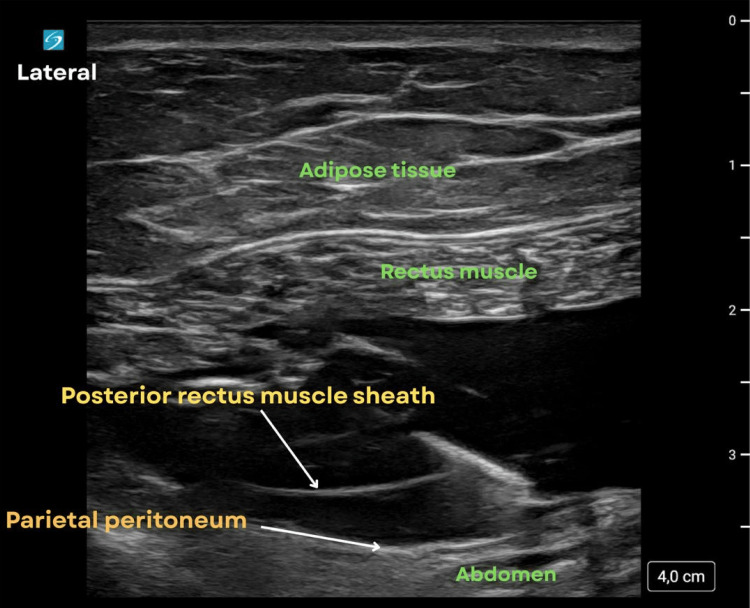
DRS block After an initial saline injection into the conventional rectus sheath plane to visualize the needle tip, the needle is advanced through the posterior rectus sheath into the preperitoneal plane. Injection of the local anesthetic opens the preperitoneal compartment, producing a characteristic V-shaped spread and displacing the parietal peritoneum deeper. Ultrasound orientation markers are shown within the image (lateral to the left, medial to the right; superficial at the top, deep at the bottom). DRS: deep rectus sheath
